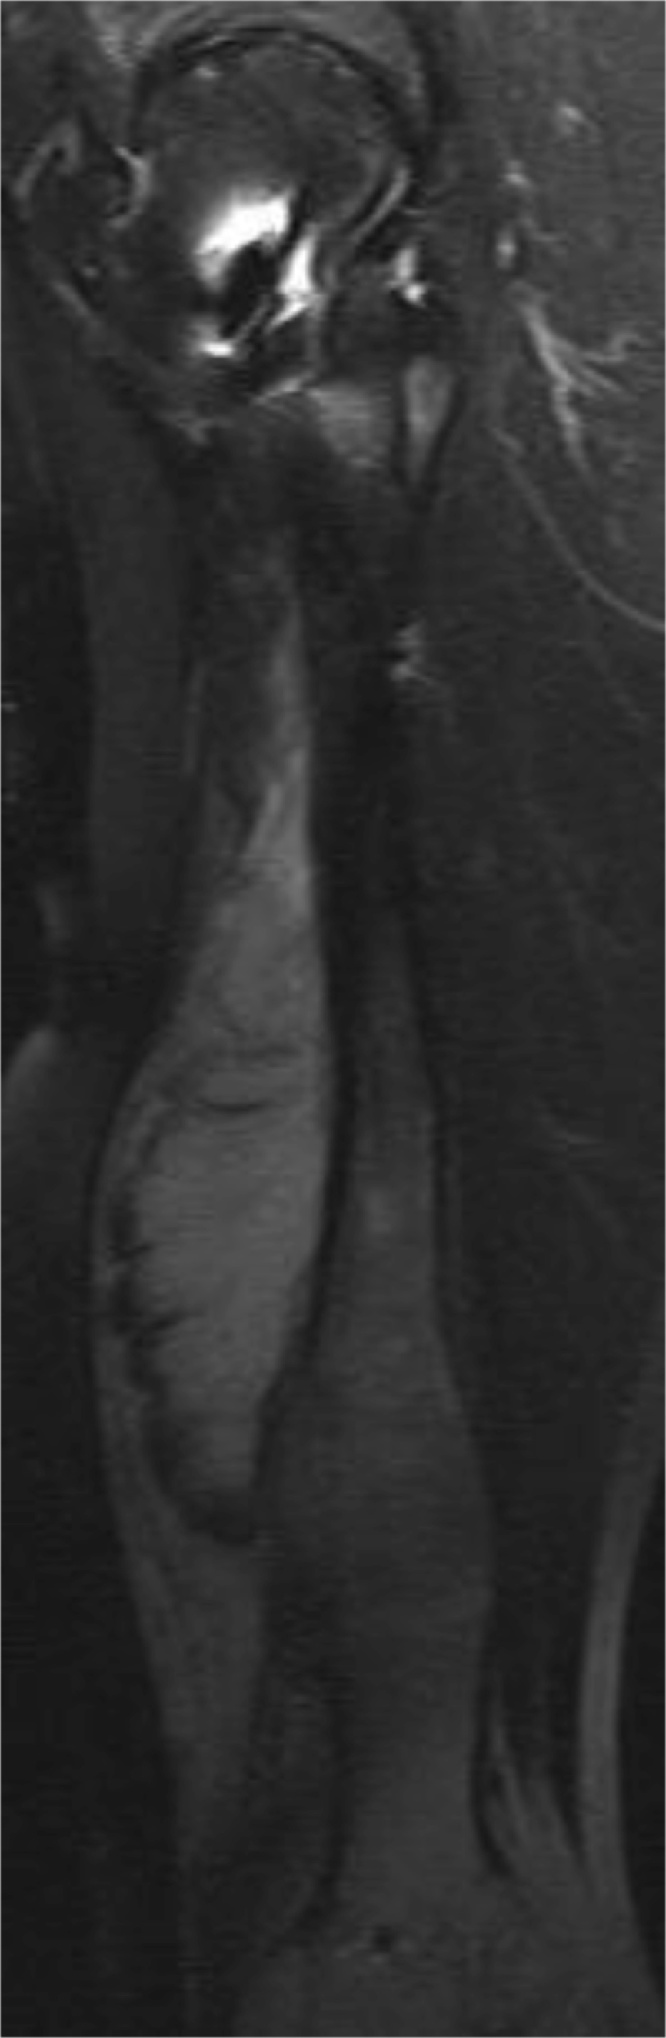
Magnetic resonance Imaging of the right femur in anterior-posterior view showing a massive irregular periosteal hypertrophic lamellar ossification at with an irregular sunray pattern. An additional infiltration of the surrounding musculature and soft tissue cannot be clearly ruled out.
